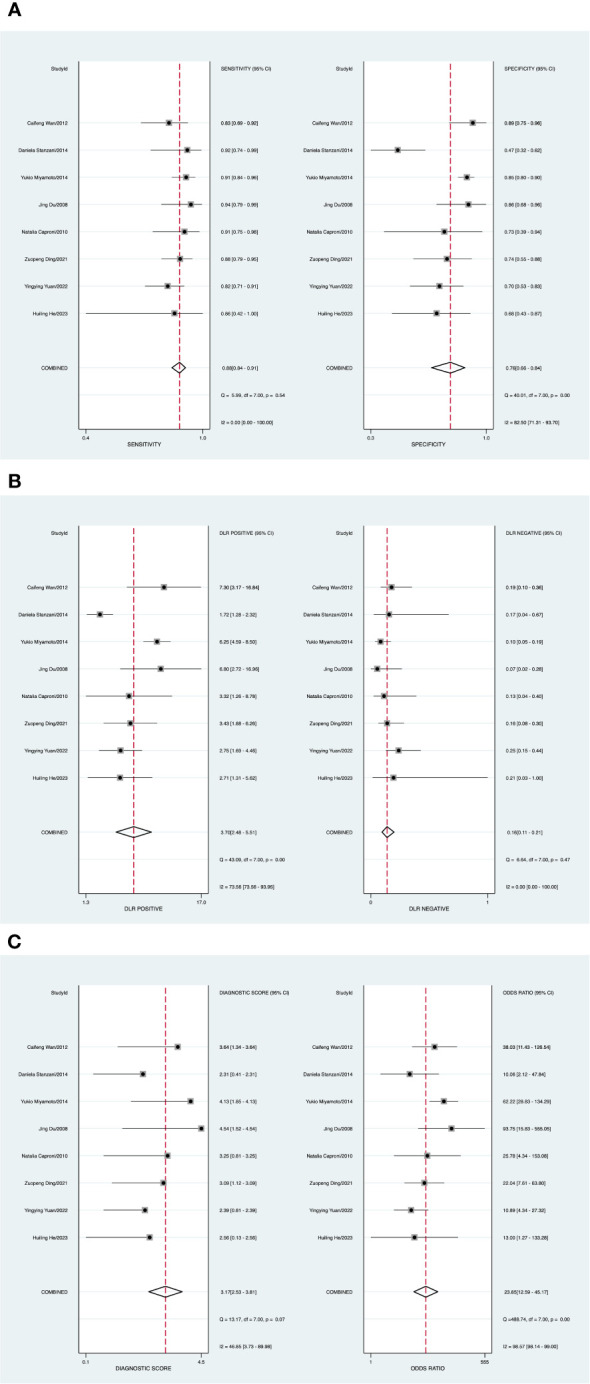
(A) Forest plot of sensitivity and specificity of contrast-enhanced ultrasound (CEUS) in the diagnosis of breast cancer. (B) Forest plot of diagnosis likelihood ratio (DLR). (C) Forest plot of the diagnostic odds ratio (DOR).
